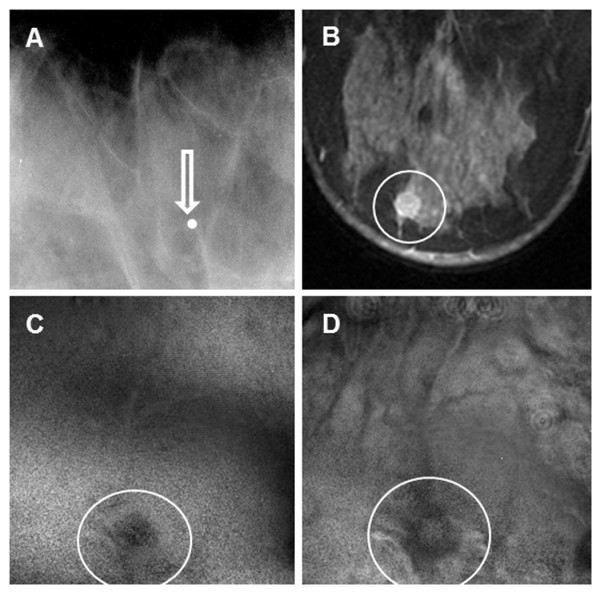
Images from case 3. (A) Mammogram shows only a marker (arrow) placed on the site of the palpable mass (no mass visible in image). (B) Magnetic resonance image shows the lesion (circle). (C-D) Vibro-acoustography images at a 2.5-cm depth (C) and 3.0-cm depth (D) show the lesion (circles).
